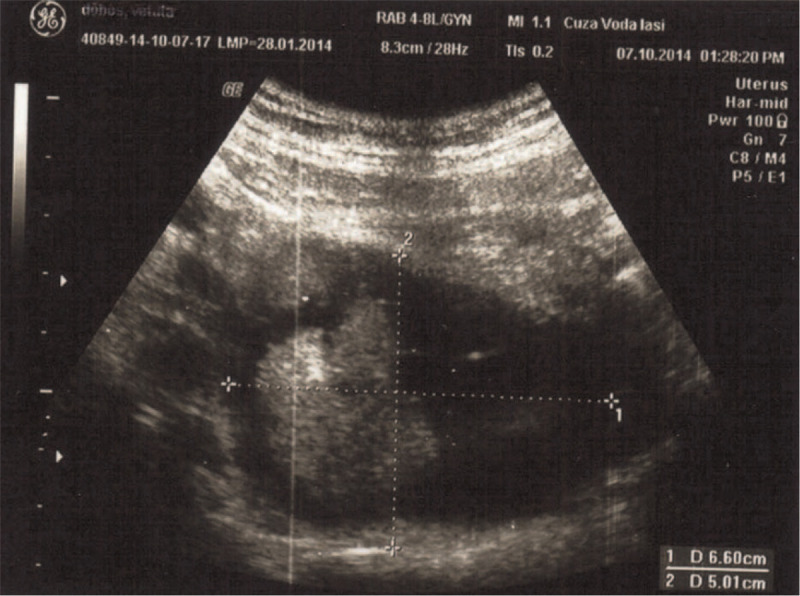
Ovarian teratoma-ultrasound image: parauterine teratoma, left ovary with a normal structure.
